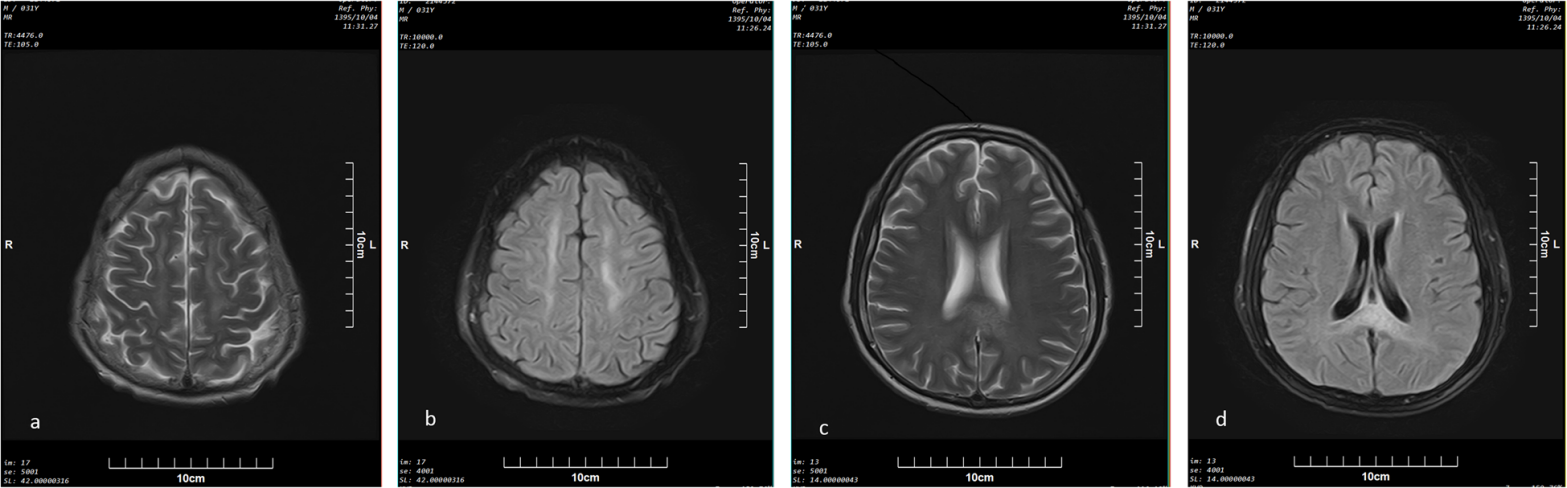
Axial (a) T2 and (b) FLAIR sequences of a 31-year-old man (patient number 5) , 12 days after presenting to emergency department with AOE symptoms, symmetric areas of hyperintensity in both centrum semiovale are seen. Axial (c) and (d) FLAIR sequences of the same patient at the mid ventricular level show hyperintensity in the splenium of corpus callosum
